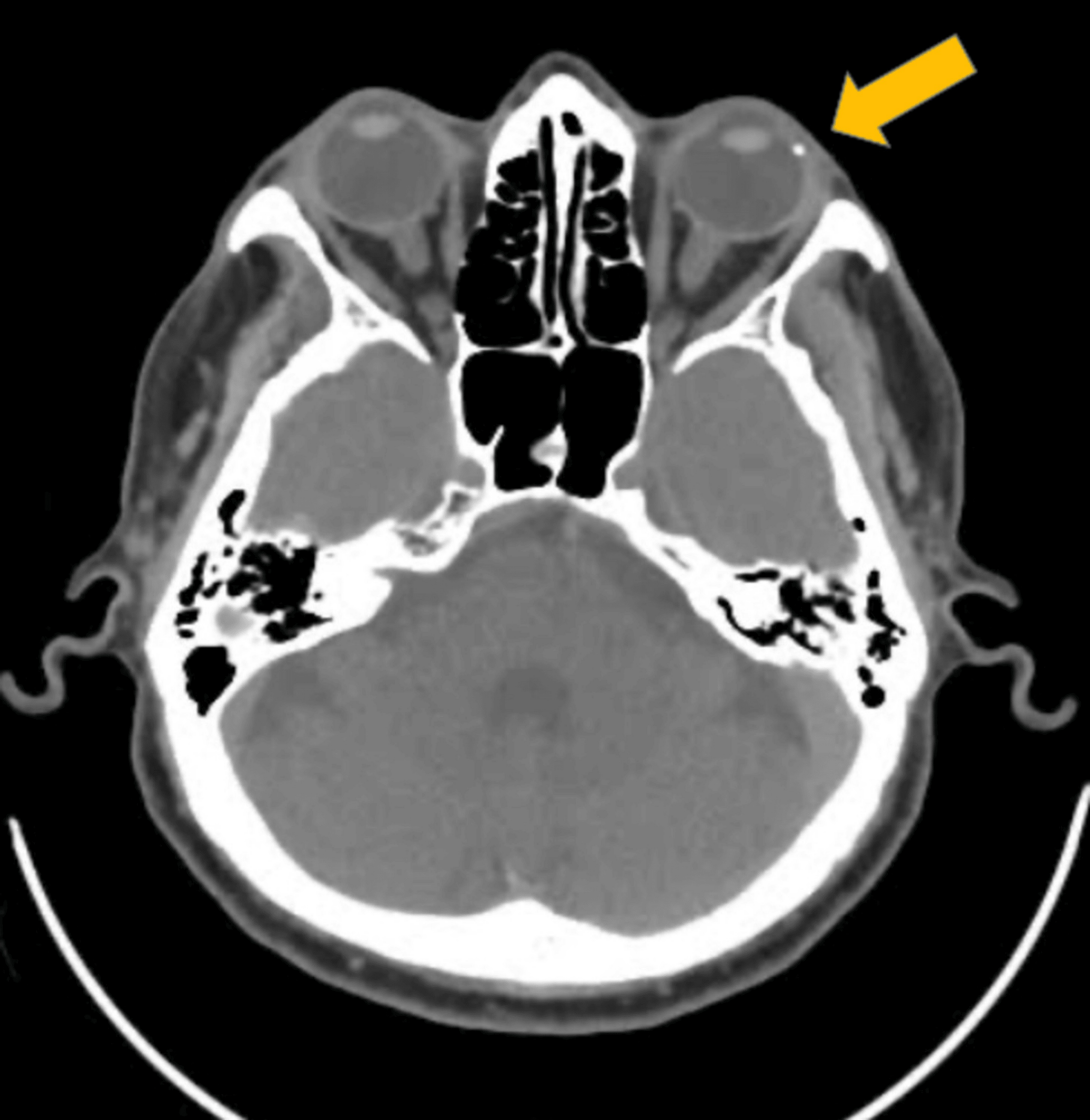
Computed tomography (CT) image showing a hyperdense lesion at the temporal globe of the left eye.
